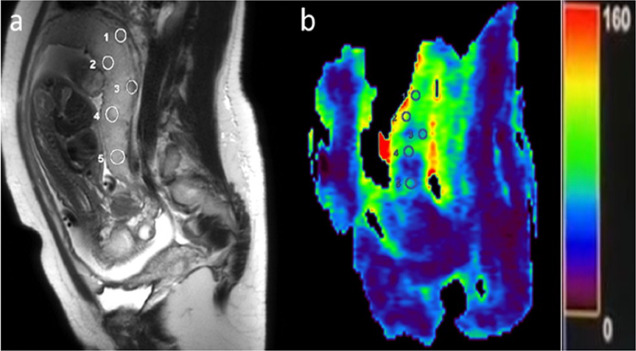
A 25-year-old female with 30 weeks of gestation was referred for placental magnetic resonance imaging (MRI) to evaluate intrauterine growth restriction (IUGR). The MRI revealed a placental blood flow value of 77 ml/min/100 g. (a) T2-weighted SSFSE sagittal image showing the placenta, (b) Placental Pattern-based Morphometry (PBM) map with the Region of Interest (ROI) marked on the placental image
